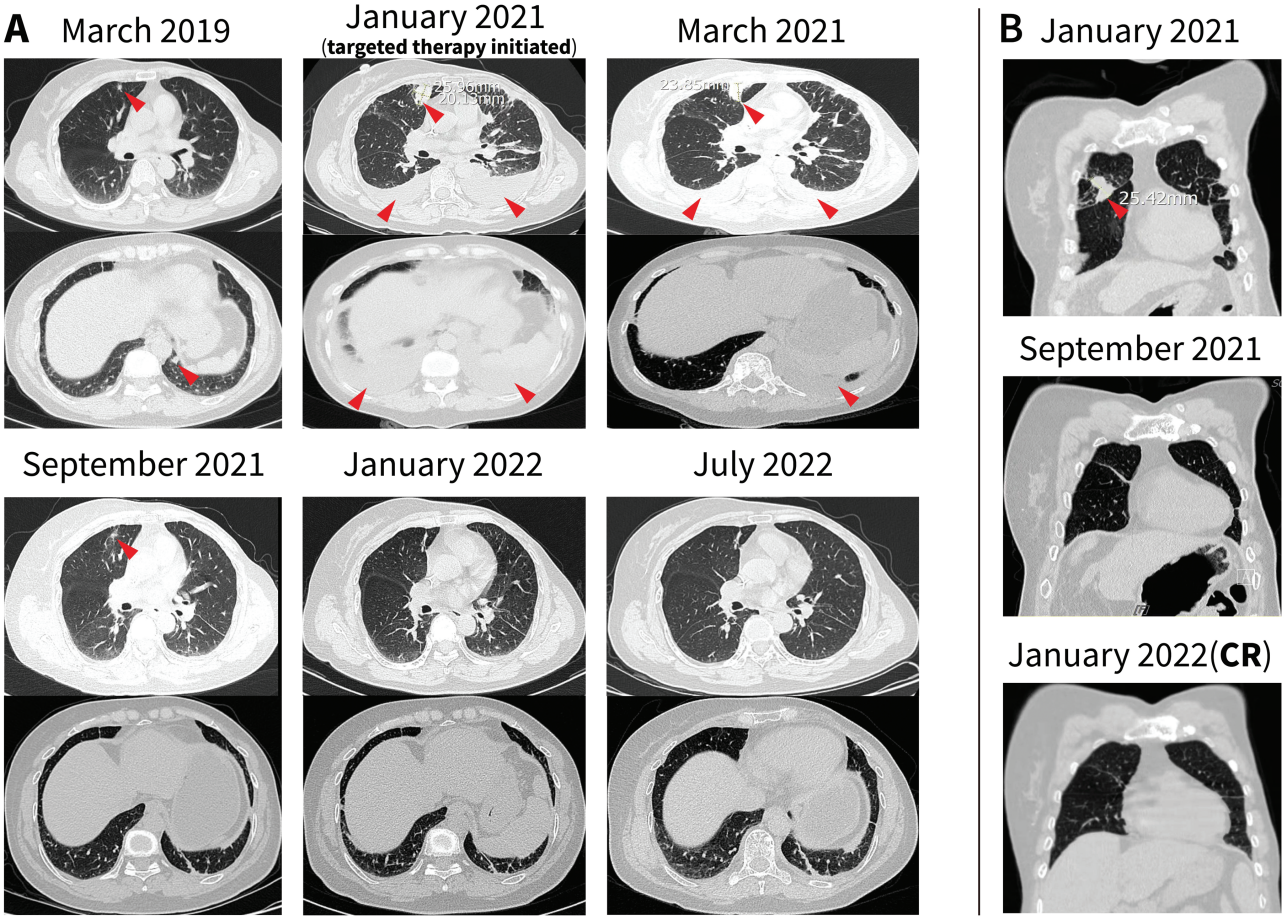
CT scans showing the responses of the lung metastases. A: Gradual partial response of the target lesion and pleural effusion in the right and left lung (arrows). B: Complete response of the target lesion in the right lung (arrows). CR, complete response; NGS, next-generation sequencing.
